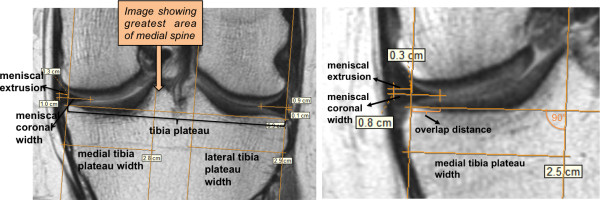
Example of measurements on mid-coronal 3 T intermediate weighted knee MR images using eFilm 3.4 software (the vertical lines are supporting lines perpendicular to the tibial plateau created to aid measurements).
